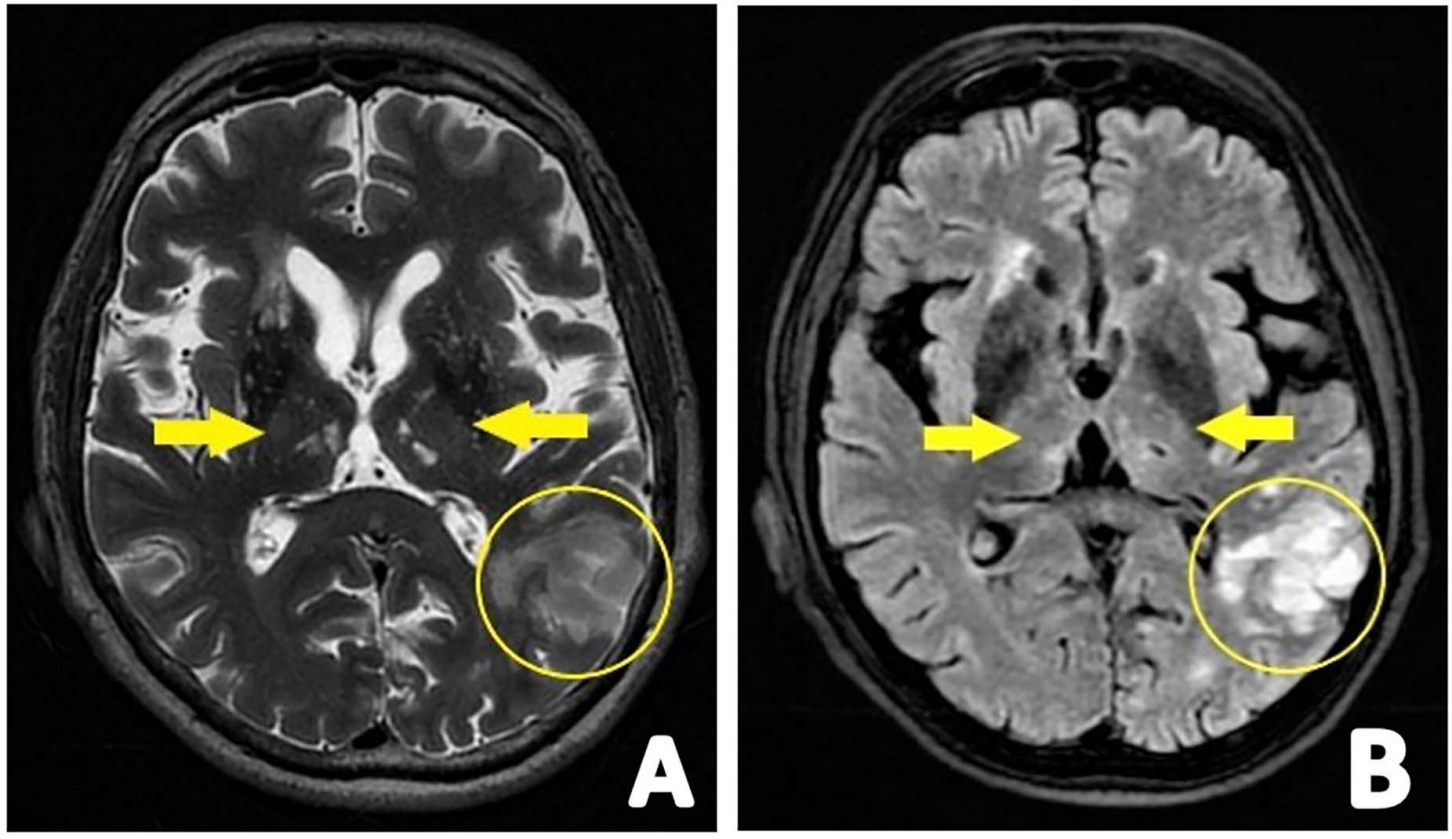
(A) Axial T2W and (B) axial FLAIR images showing strategic infarctions in both thalami and in the parieto-occipital watershed area. Images were obtained in a male patient, age 71, with clinical manifestation of vascular dementia. FLAIR: Three-dimensional T2-weighted fluid-attenuated inversion-recovery imaging.
